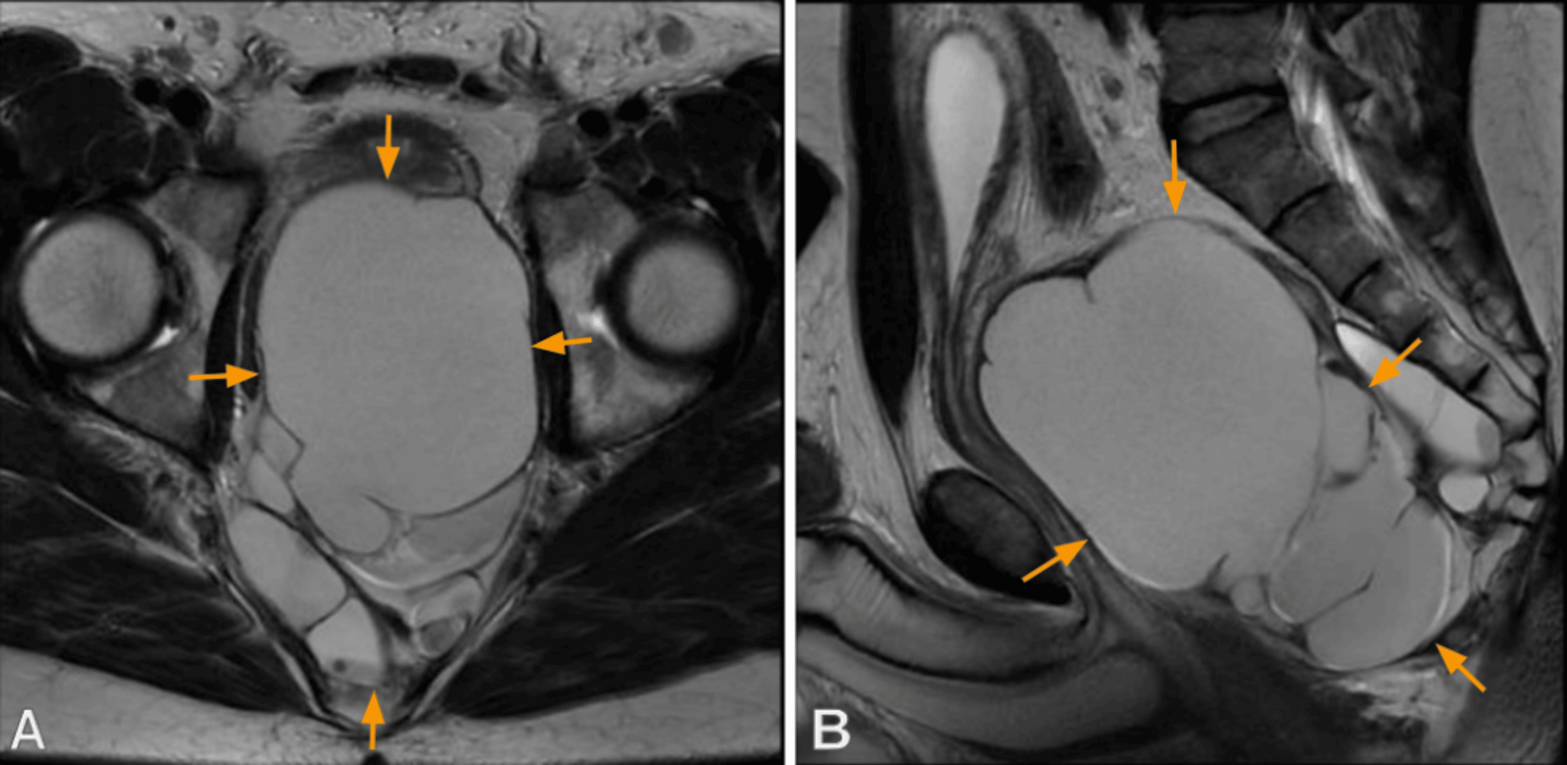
MRI showing (A) axial T2-weighted image and (B) sagittal T2-weighted image The image shows a large, multiloculated cystic mass in the pelvic cavity, with thin, enhancing septa and some parietal calcifications, as well as fluid-filled loci with a high protein content. The lesion pushes against the remaining pelvic organs, without invading them. These findings supported the hypothesis of lymphangioma, measuring 156 × 95 mm (longitudinal (L) × anteroposterior (AP)).
